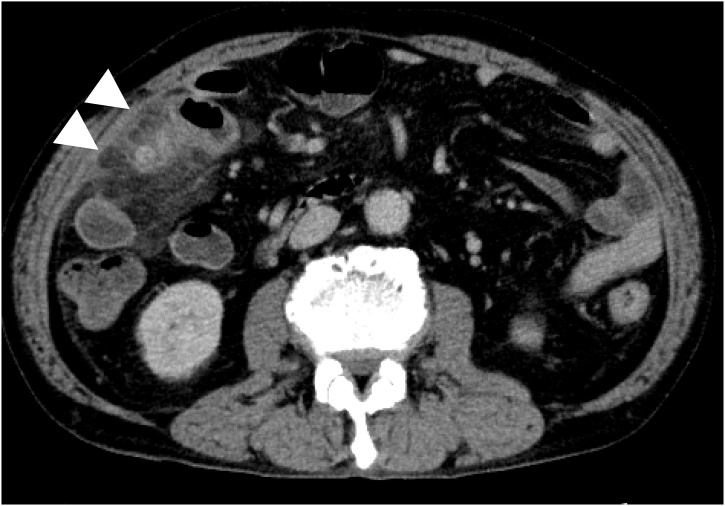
CT showed an enhanced mass of 20 mm diameter that continued to the wall of ileum. A fat-dense area was enhanced around the mass.
